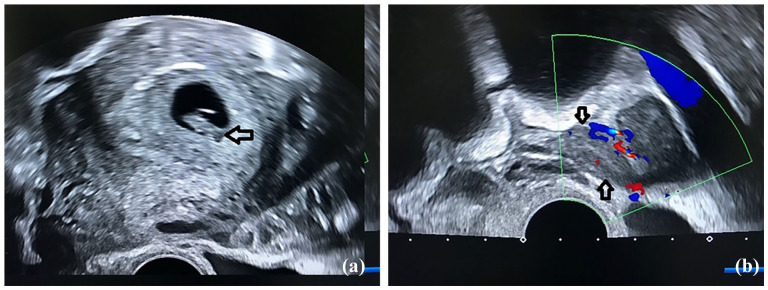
(a) Transvaginal ultrasonography (TVUS) revealed a single intrauterine (IU) gestation with a crown–rump length (CRL) of 12 mm (Black arrow) with a positive fetal heart rate and (b) A suspected ectopic gestation (Black arrows) in the left fallopian tube with positive Doppler ultrasound peripherally.
